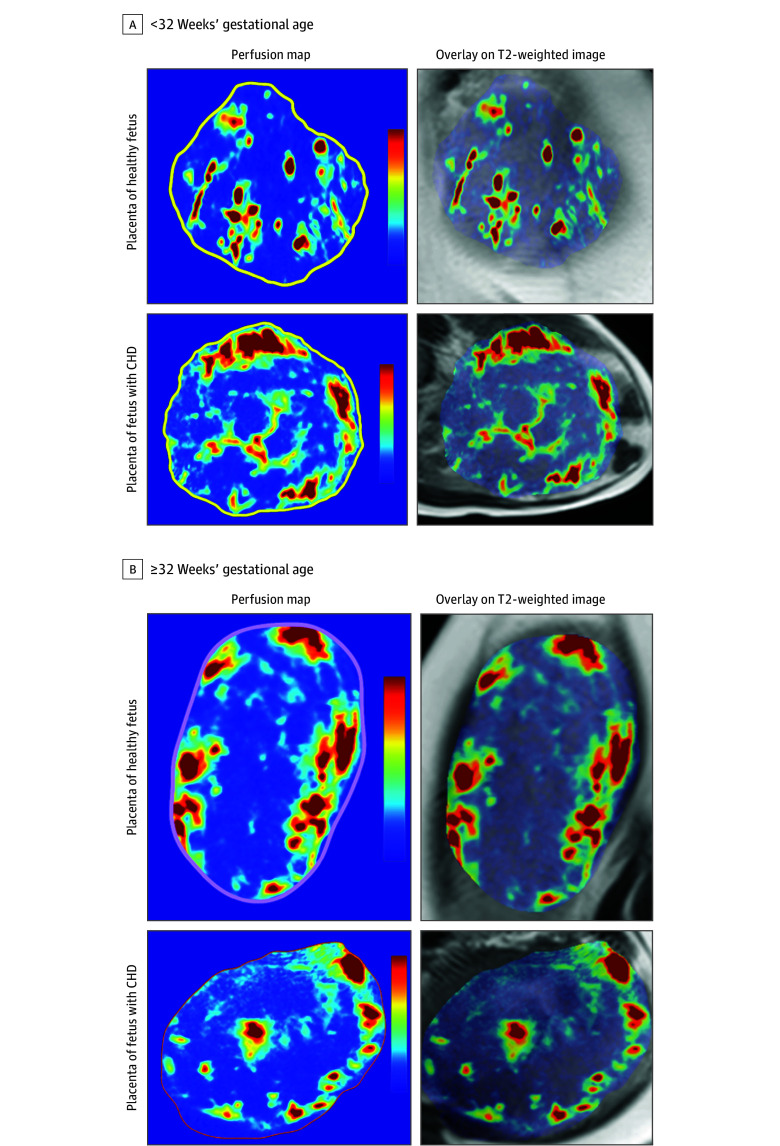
Representative Placental Perfusion Maps A, Representative quantitative perfusion maps (left) and perfusion maps overlaid on anatomical T2-weighted images (right) from an individual with a fetus without congenital heart disease (CHD) at 28 weeks 5 days’ gestation (top row) and an individual with a fetus with CHD (tetralogy of Fallot) at 29 weeks 2 days’ gestation (bottom row). B, Similar representative images from an individual with a fetus without CHD at 35 weeks 5 days’ gestation (top row) and an individual with a fetus with CHD (coarctation of the aorta with right-to-left ventricular size discrepancy, mild ventricular dilation, and hypertrophy) at 36 weeks’ gestation (bottom row). Color scale indicates perfusion values from 0 to 300 mL/min/100 g.
